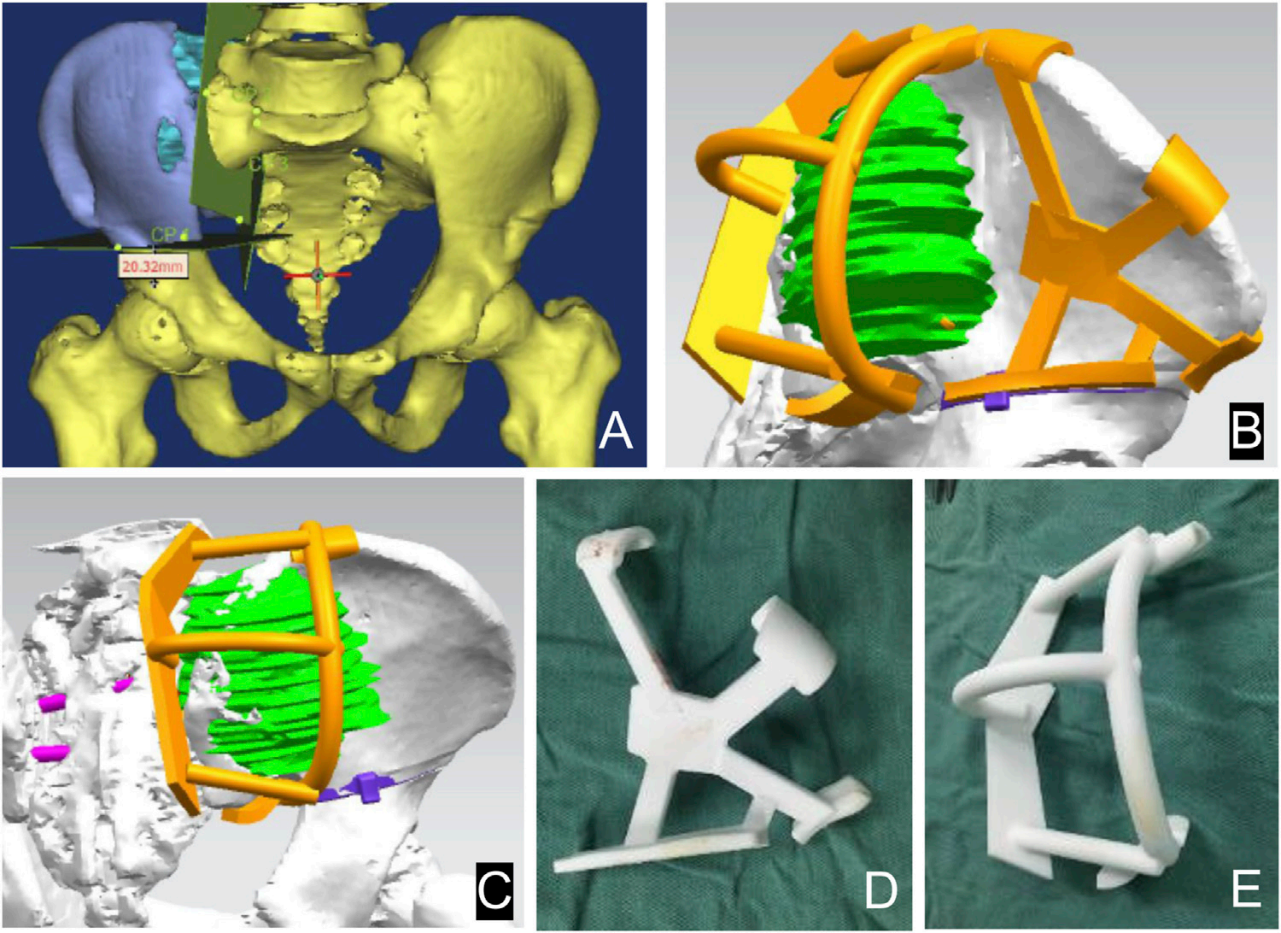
Design of the 3D bone tumor model and cutting guide. The 3D bone tumor model created from CT data was used for surgical planning (A). The personalized cutting guide designed by computer (B, C). External view of the cutting guide manufactured by 3D printing technology (D, E).
